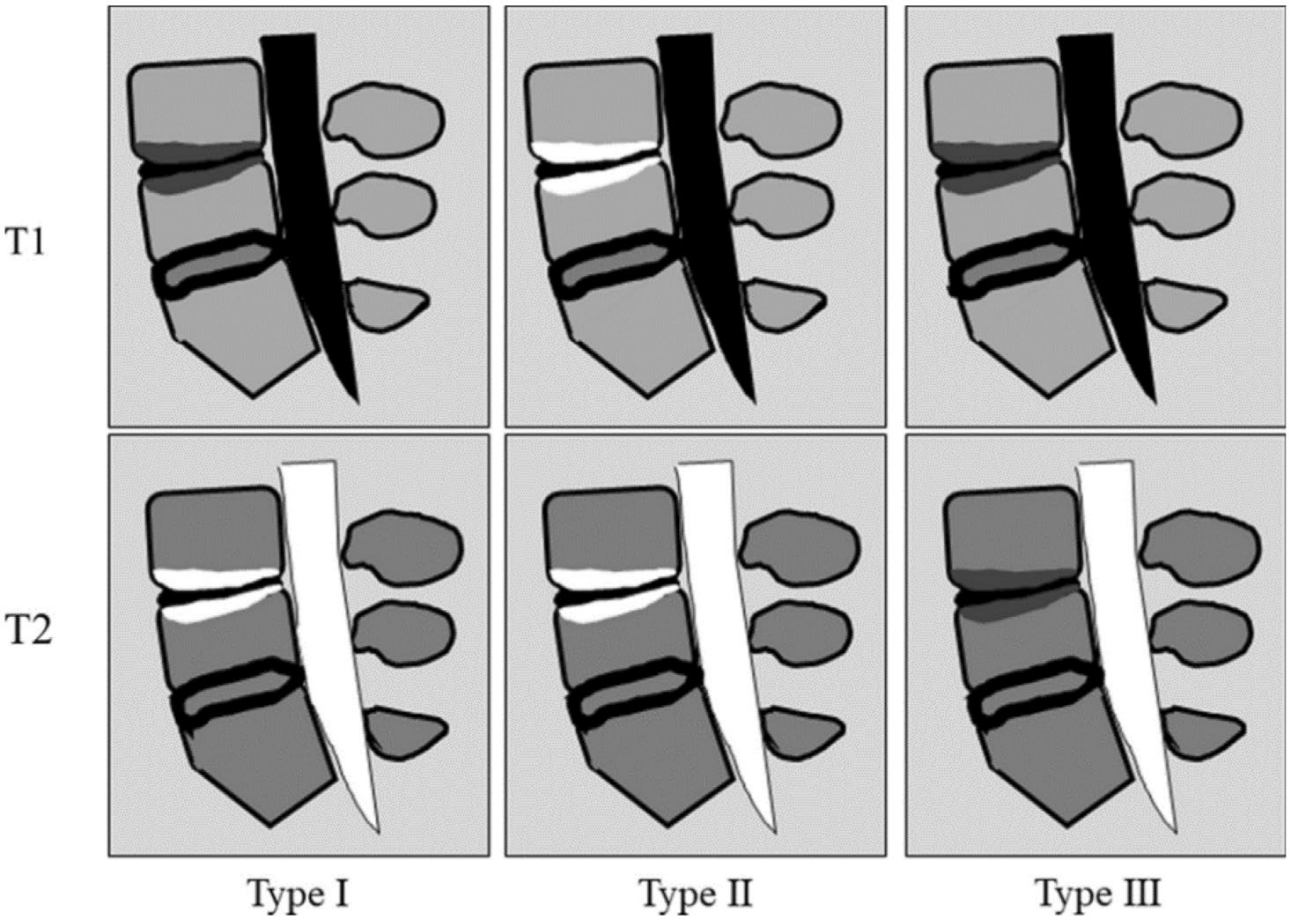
Sagittal magnetic resonance images showing types I, II, and III Modic changes. Type I: Low intensity on T1‐weighted images (T1WI), high on T2‐weighted images (T2WI). Type II: High intensity on both T1WI and T2WI. Type III: Low intensity on both T1WI and T2WI. Retrieved from Manabe et al. [48].
